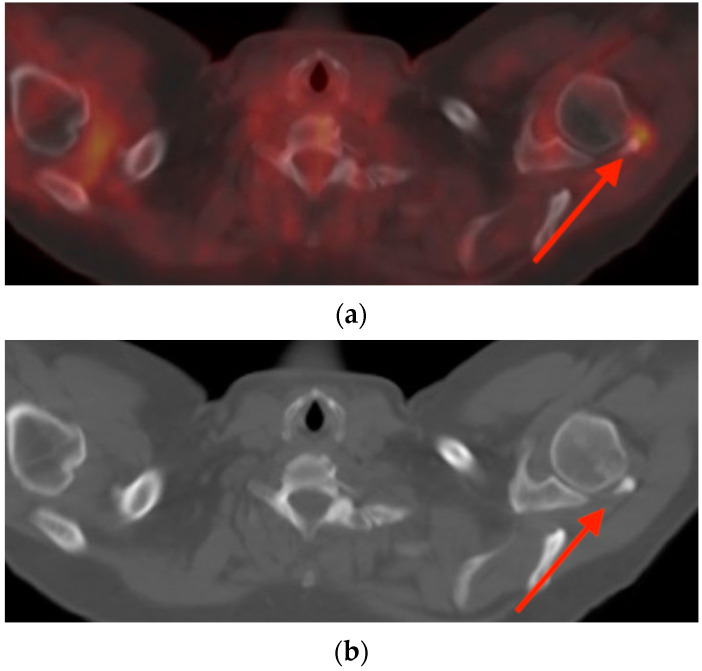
(a) Axial fused FDG PET/CT images in a 52-year-old woman with follicular lymphoma demonstrate a hypermetabolic focus adjacent to the posterolateral aspect of the left humeral head, with SUVmax 2.5 (red arrow). (b) Plain axial CT images better depict the amorphous calcification within the rotator cuff tendons (red arrow). The findings are consistent with calcific tendinopathy of the rotator cuff with associated inflammatory reaction within the surrounding tissues. CT = computed tomography. FDG PET/CT = fluorine-18 fluorodeoxyglucose positron emission tomography/computed tomography. SUVmax = maximum standard uptake value.
